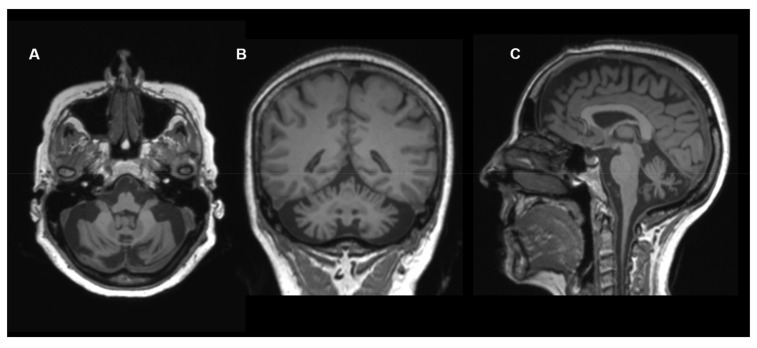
Neuroimaging in COQ8A deficiency: Brain MRI (T1-weighted images, (A) axial view; (B) coronal view; (C) sagittal view) of a 60-year-old female with COQ8A deficiency. Images show cerebellar atrophy. Other MRI images of this individual were published previously [45].
